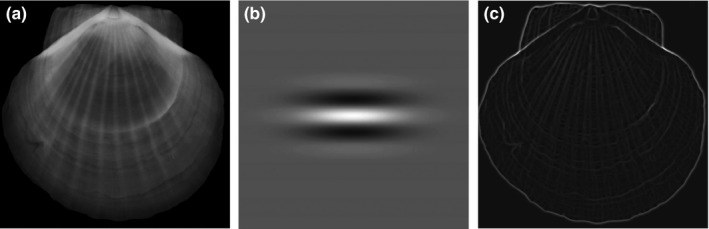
Enhancement of CT image: (a) CT image of scallop; (b) two‐dimensional Gaussian kernel; (c) enhanced image by matched filtering with Gaussian kernel (MFGK)
